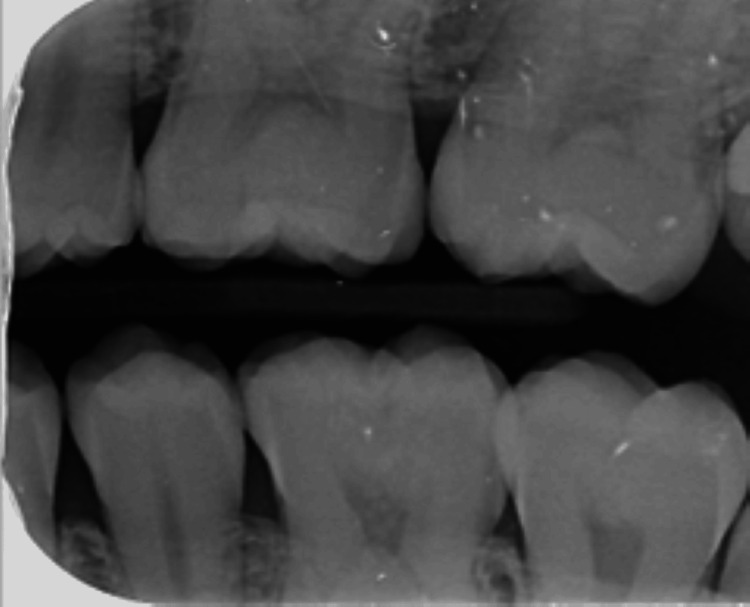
Bitewing radiograph of left quadrants.
